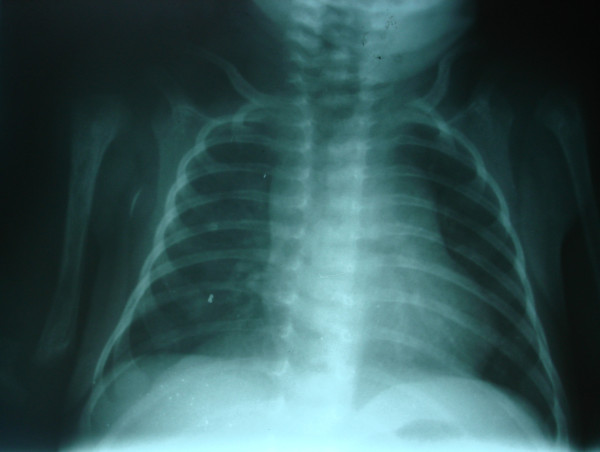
Chest X-ray showing complete resolution of symptoms at the end of anti-biotic therapy.
